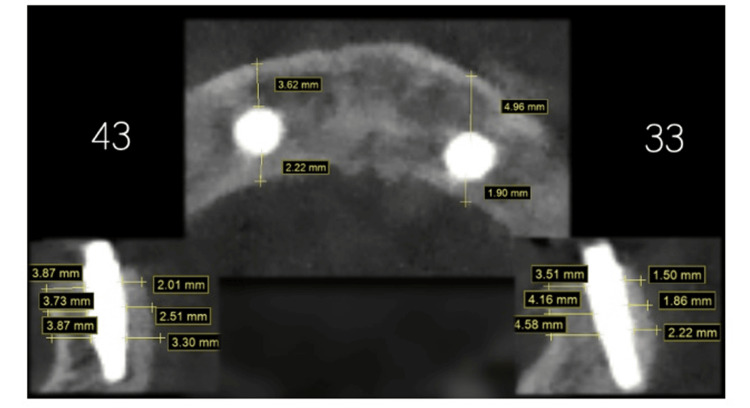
Postoperative cone-beam computed tomography confirming the three-dimensional position of the new intraforaminal implants and the absence of peri-implant radiolucency.
